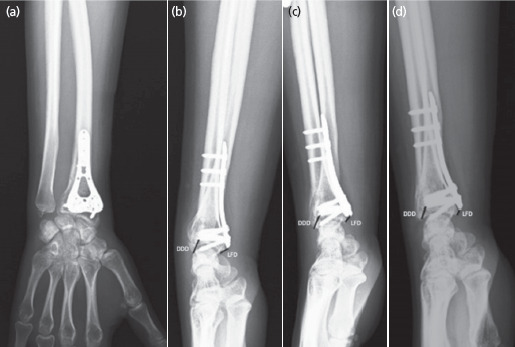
Method of measuring the radiographic parameters of the distal radius fractures: Radial collapse was observed in the radiographs taken during the one-year follow-up period. (a, b) Antero-posterior and lateral view at the time of discharge. (c) Lateral radiograph after three months. (d) Lateral radiograph at one year follow-up.
